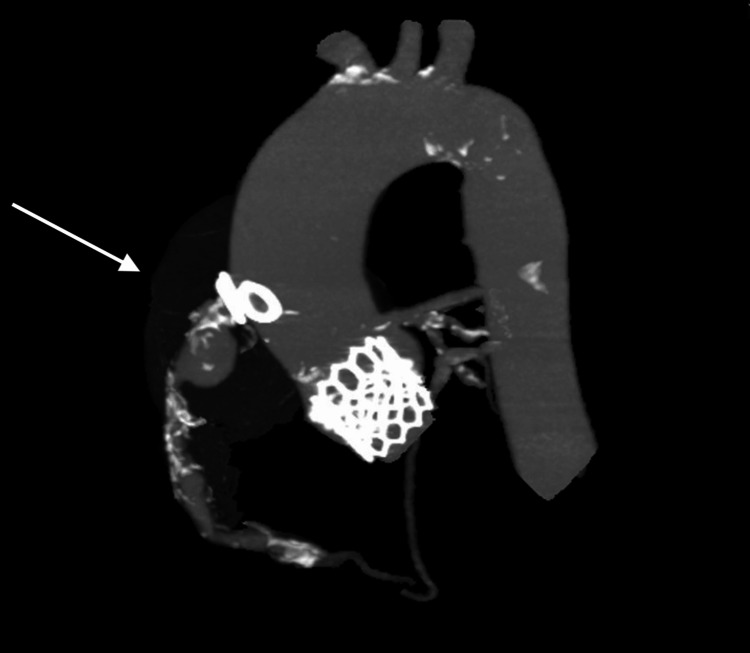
Pre-stent CT image highlighting SVG-RCA pseudoaneurysm Computed tomography scan showing a saphenous vein graft (SVG) to right coronary artery (RCA) pseudoaneurysm. The aortic-ostia diameter by the CT measured ~6.5 x 6 mm. A total length of 150 mm from the proximal to distal end of the pseudo-aneurysmal segment was needed to effectively cover and seal the pseudoaneurysm.
